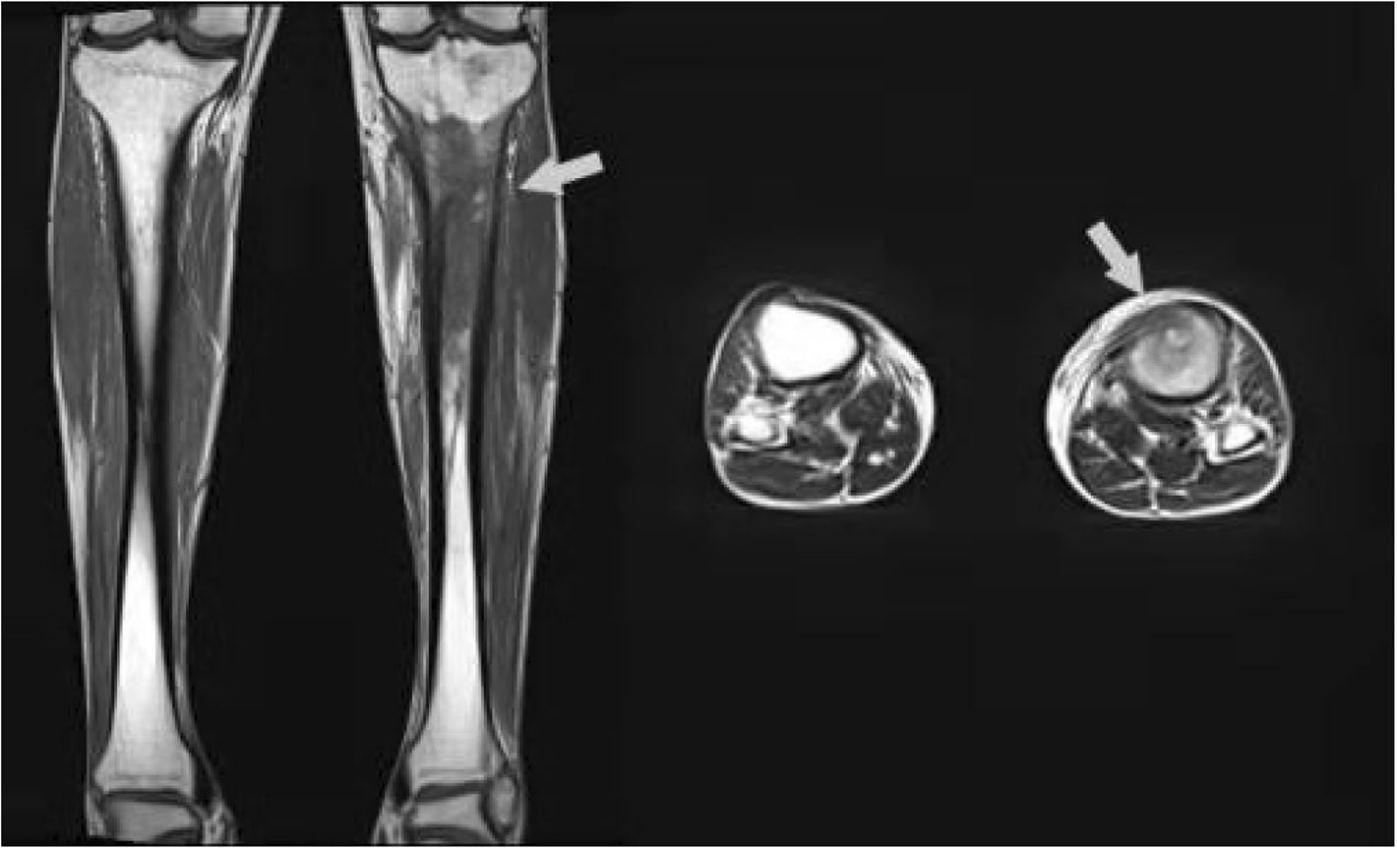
Preoperative MRI examination significantly assisted to define the diagnosis of C-M type III osteomyelitis and determine the extent of debridement
